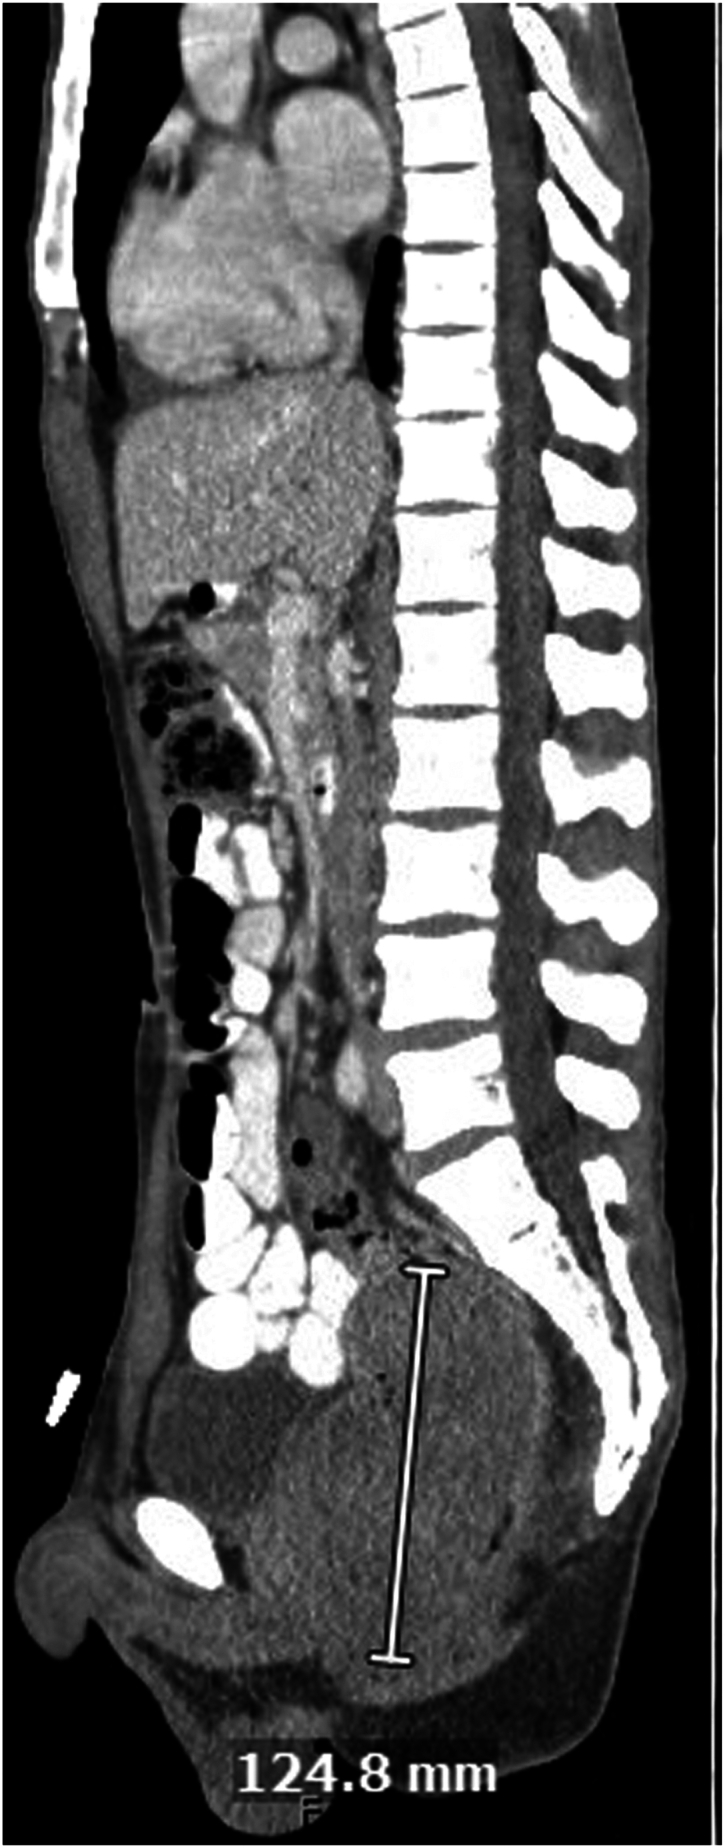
CT scan, coronal view showing large rectal mass.
